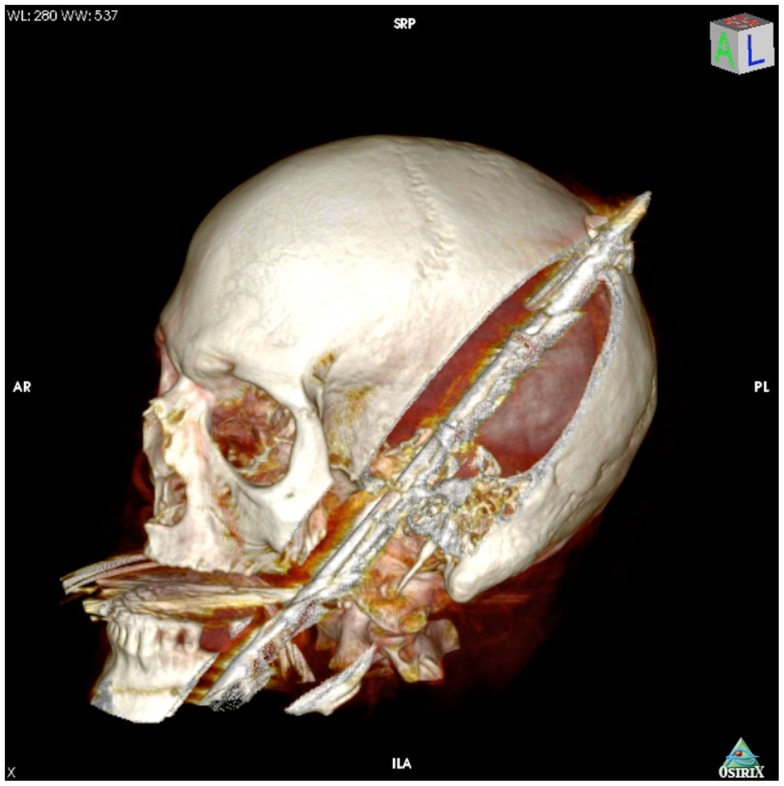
3-dimensional computed tomography reconstruction of the spear’s tract through the oropharynx and skull.
